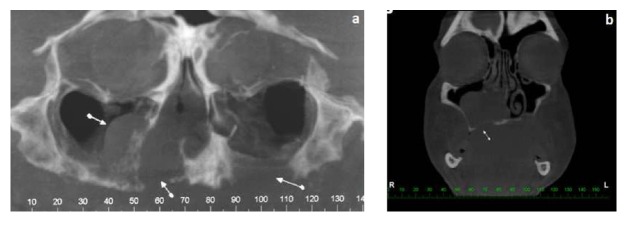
Extent of bone destruction seen on CT scans ((a) CT, axial view; (b) CT, coronal view).
